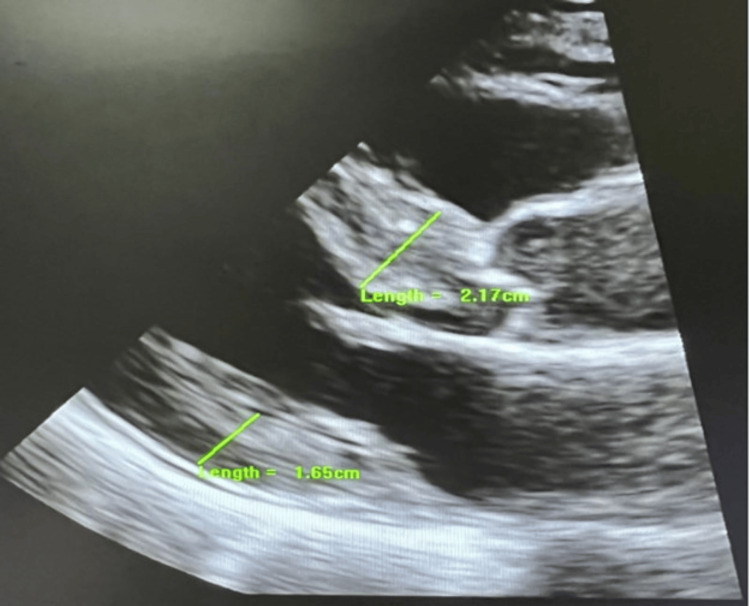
Transthoracic echocardiogram in a parasternal view showed severe left ventricular hypertrophy, which measures 2.17 cm in the basal septum and 1.65 cm in the basal left ventricular posterior wall.
